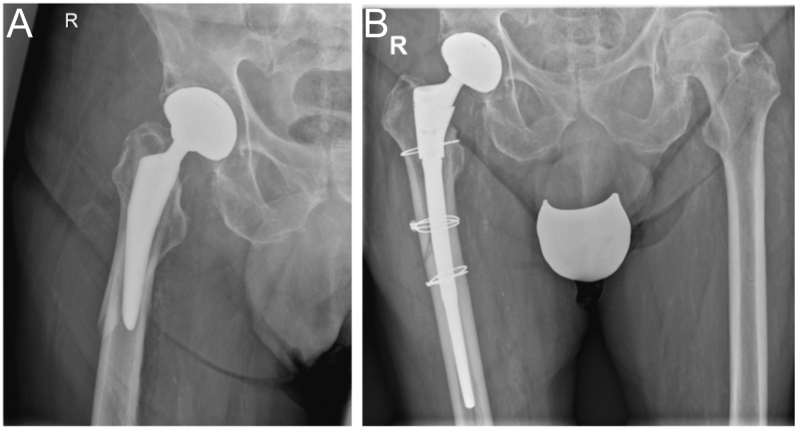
(A) Preoperative and (B) postoperative anteroposterior radiographs of the pelvis of the patient exhibited in Fig. 1, six weeks after index THA surgery for FNF. The patient presents to the emergency department because of right hip pain after a fall from his height. A periprosthetic fracture Vancouver B2 was diagnosed and was treated with a dual mobility revision arthroplasty.
